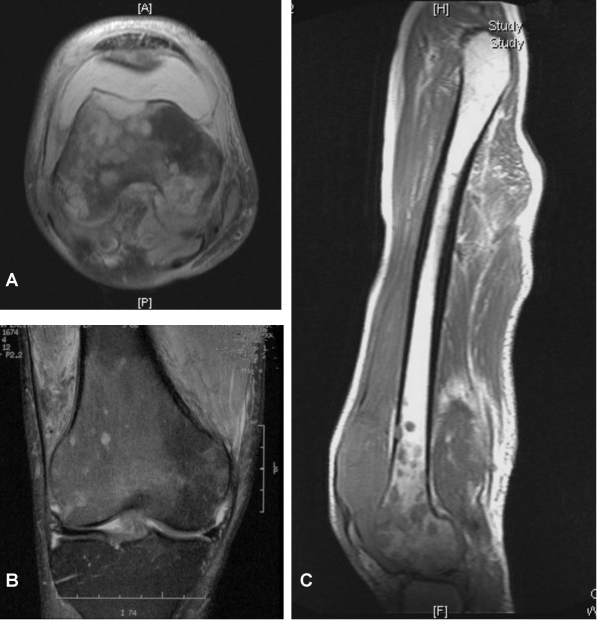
MRI demonstrating extensive medullary signal abnormality over the distal femur and proximal tibia with a large haemarthrosis. (A) T1-weighted axial, (B) T2-weighted coronal and (C) T1-weighted sagittal views of the lesion.
